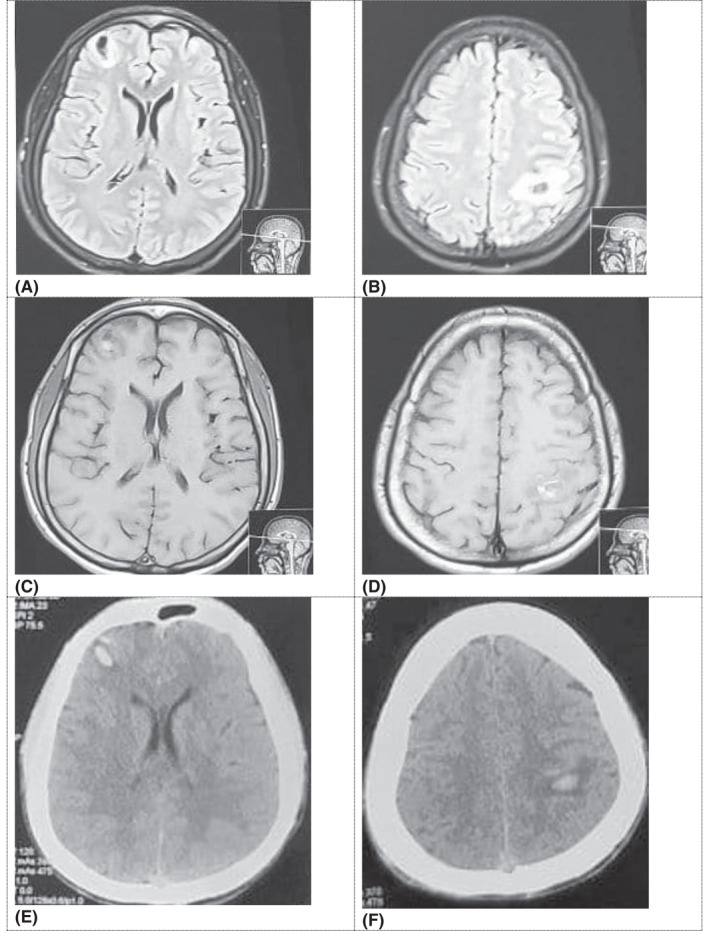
(A, B) FLAIR Axial images shows central hypointense with surrounding hyperintense lesion in right frontal and left parietofrontal region; (C, D): T1‐weighted axial shows central hyperintense with surrounding hypointense lesion in right frontal and left parietofrontal region: (E, F) CT head plain shows central hyperdense with surrounding hyperdense lesion in right frontal and left parietofrontal region
